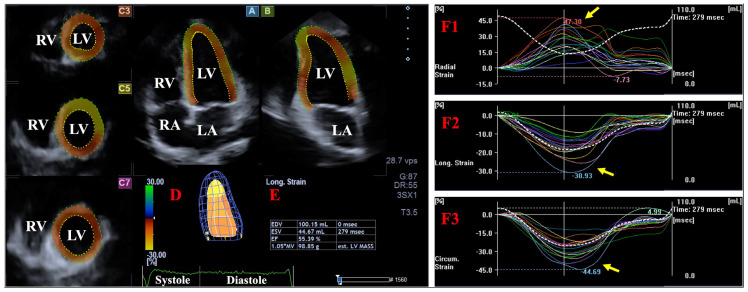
Visual representation of the imaging planes employed in the evaluation: apical four-chamber (A) and two-chamber (B) long-axis views, along with short-axis cross-sections at the apical (C3), midventricular (C5), and basal (C7) regions of the left ventricle (LV). Panel (D) shows the virtual three-dimensional reconstruction of the LV generated by the software, while the related volumetric parameters are displayed in Panel (E). Panels (F1–F3) present the global (white) and segmental (colored) time-strain curves for radial, longitudinal, and circumferential LV deformation, respectively, alongside the time–LV volume change curve (dashed white curve). Yellow arrow represents maximum of the LV strains at end-systole. Abbreviations: EDV: end-diastolic volume; EF: ejection fraction; ESV: end-systolic volume; RA: right atrium; RV: right ventricle; LA: left atrium; LV: left ventricle.
